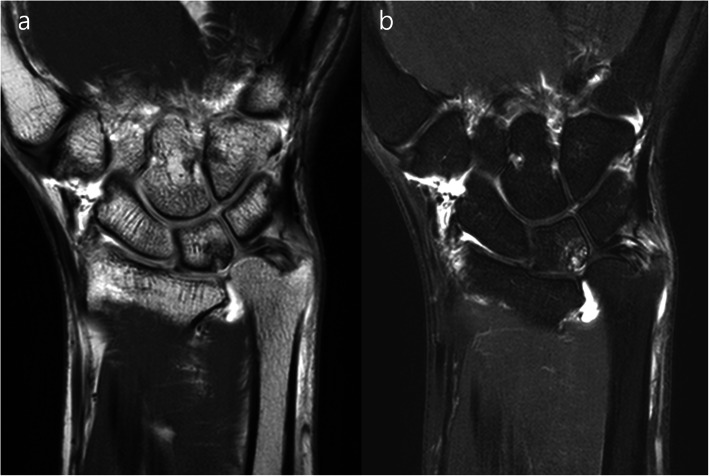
The magnetic resonance images of ulnar impaction syndrome (a T1-weighted image, b T2-weighted image on the coronal). They typically contain degenerations in an ulnocarpal joint (TFCC wear or perforation, perforation of a lunotriquetral ligament, and chondromalacia or cartilage denudation or bony edema or erosion of lunate or triquetrum or ulnar head)
